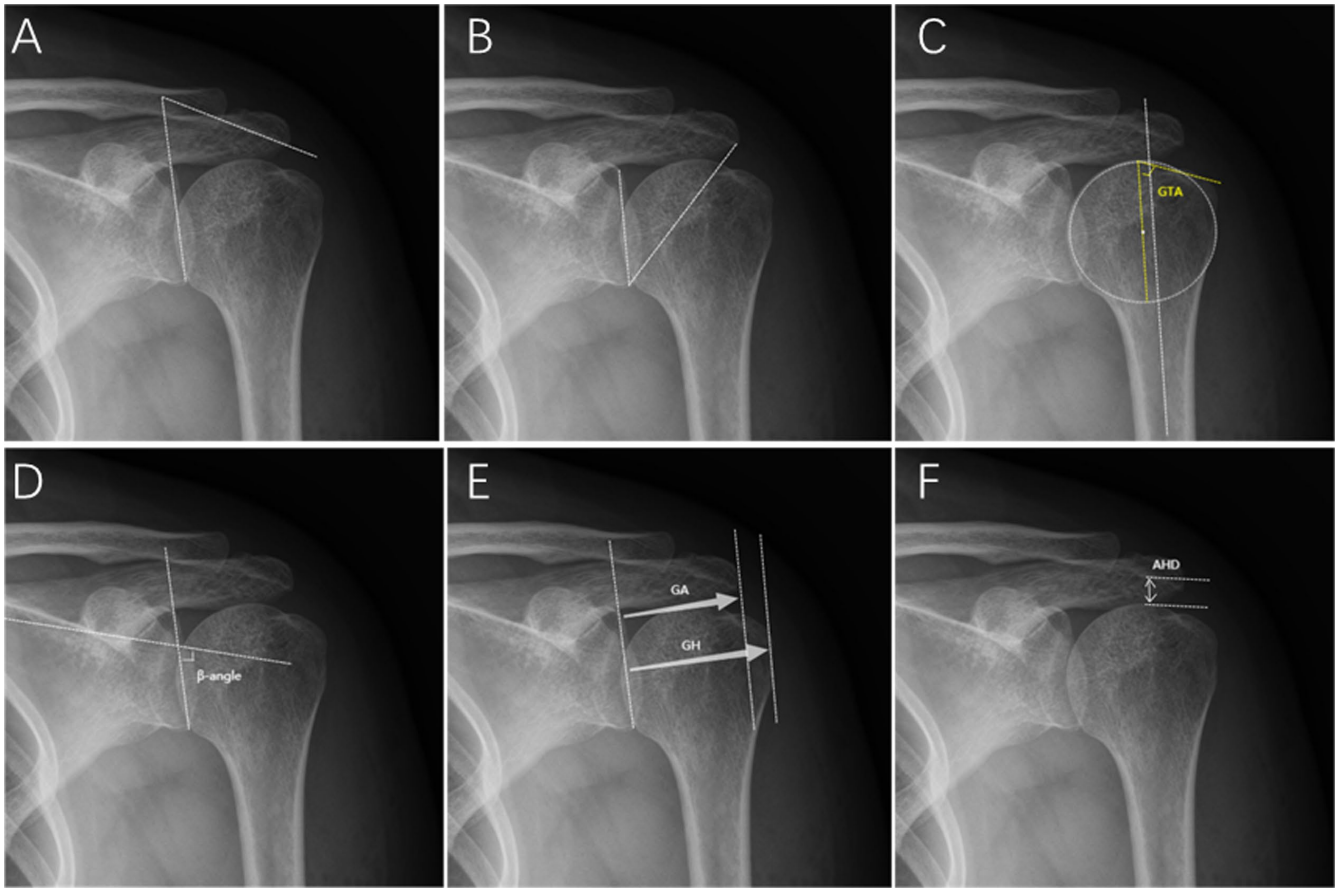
Radiographic measurements on the true anteroposterior radiograph. (A) Lateral acromial angle (LAA). (B) Critical shoulder angle (CSA). (C) Greater tuberosity angle (GTA). (D) β-angle. (E) Acromion index (AI): GA/GH. (F) Acromiohumeral distance (AHD).
